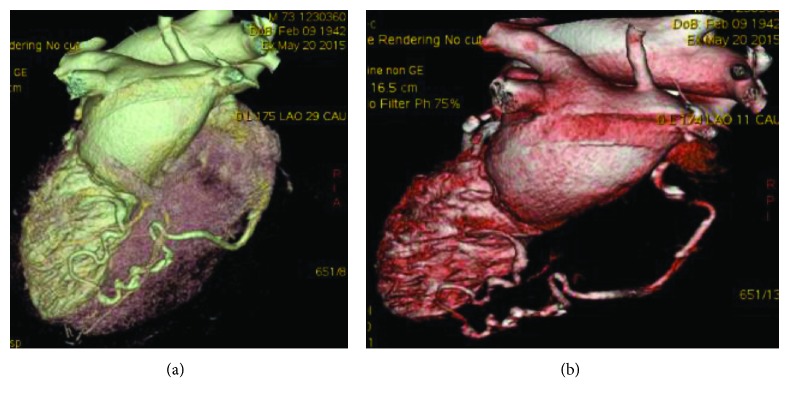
Three-dimensional (3D) reconstruction of the right coronary artery and fistula using computed tomography angiography.
